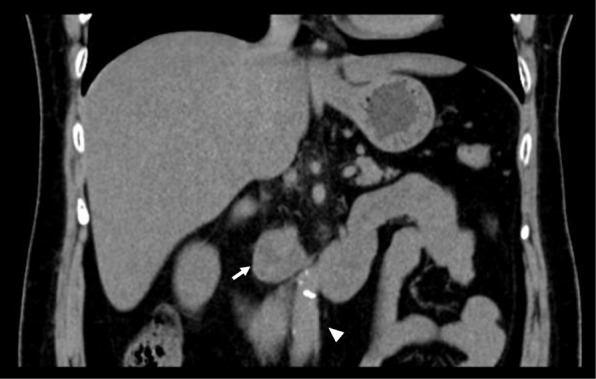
Coronal noncontrast computed tomography image. A heterogeneous mass in the right retroperitoneal region (white arrow) adjunct to the inferior vena cava (white arrow head) is demonstrated
